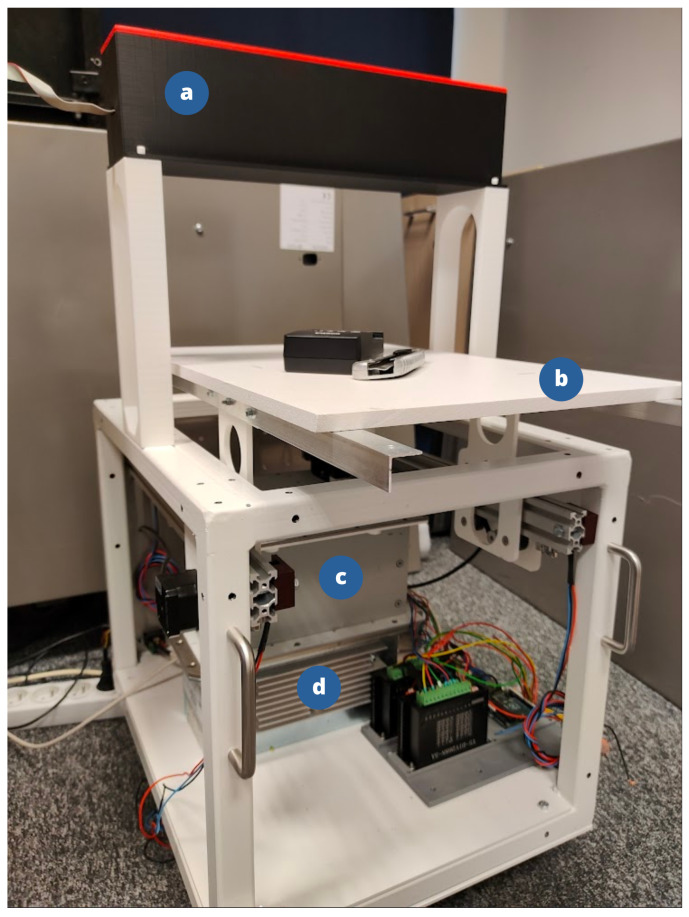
The experimental setup: (a) 3D-printed detector array. (b) Mobile platform. (c) Collimator. (d) X-ray tube.
